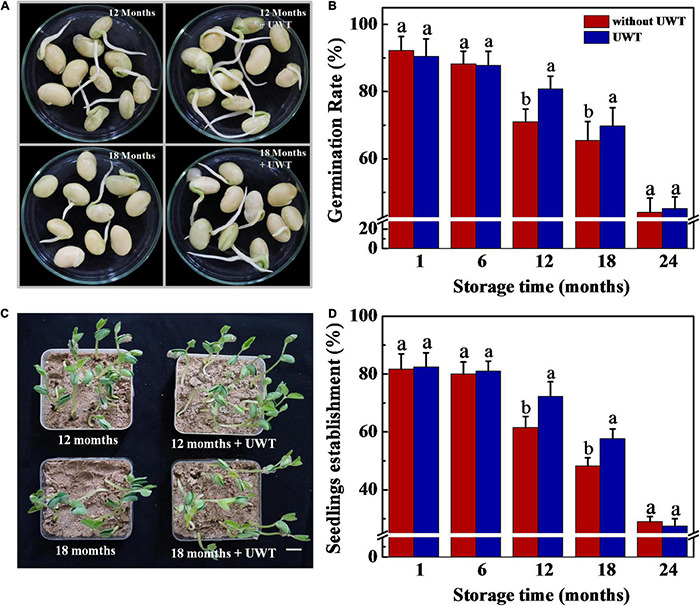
Ultrasonic waves treatment promoted germination and seedling establishment of NAT soybean seeds. (A) Images of soybean seeds at 3 days of germination. Scale bar, 10 mm. (B) Germination rates of soybean seeds at 5 days of germination. (C) Images of seedling emergence from soybean seeds at 5 days post-sowing. Scale bar, 600 mm. (D) Seedling emergence rates of soybean seeds at 5 days postsowing. Soybean seeds stored for 1, 6, 12, 18, and 24 months were used for analysis. NAT, naturally aging treatment; UWT, ultrasonic waves treatment. Different lowercase letters indicate significant differences between different treatments (*p* < 0.05).
